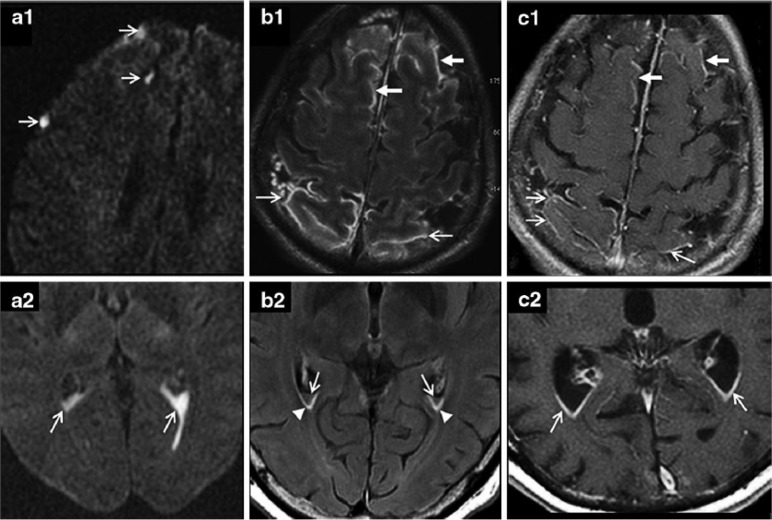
Diffusion-Weighted Imaging (DWI) (a), Fluid Attenuated Inversion Recovery (FLAIR) (b), and contrast-enhanced T1-weighted images and (c), in acute bacterial meningitis On DWI, pus is visualized in sulci (a1, thin arrows) and intra-ventricles (a2, thin arrows) as diffusion restriction. On FLAIR sequence increased signal is seen in sulci of the frontal (b1, thick arrows) and parietal (b1, thin arrows) lobes, and intra-ventricles (b2, thin arrows) and sub-ependymal space because of protein enrichment (b2, arrowheads). Both leptomeningeal and ependymal gadolinium - enhancement in T1sequence in frontal (c1, thick arrows) and parietal (c1, thin arrows) lobes (Lummel et al., 2014).
